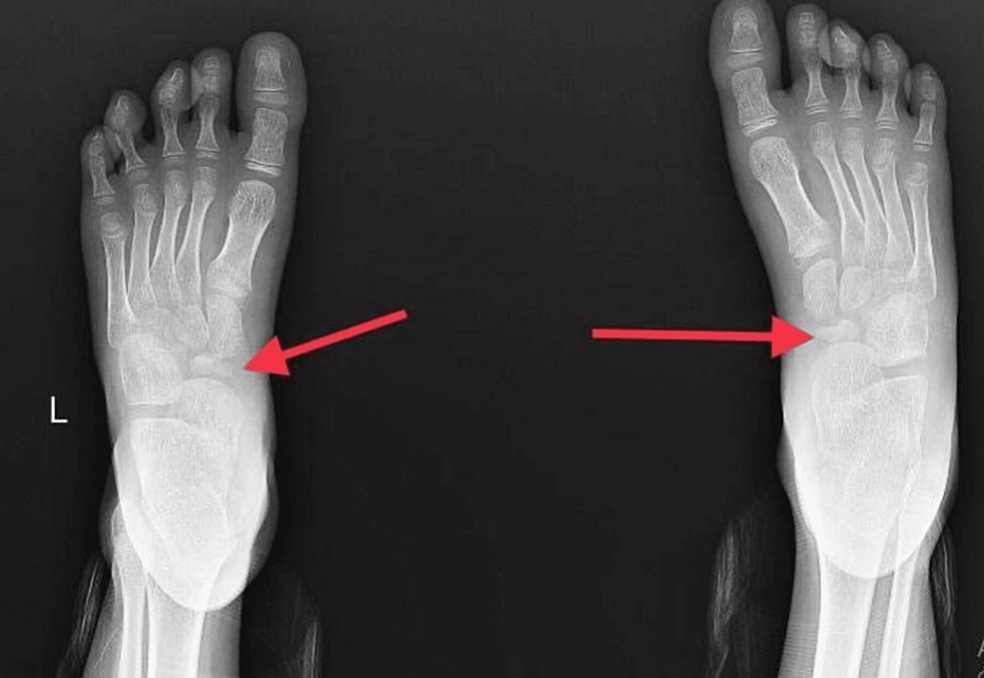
X-ray anteroposterior view of the left and right feet and ankle
